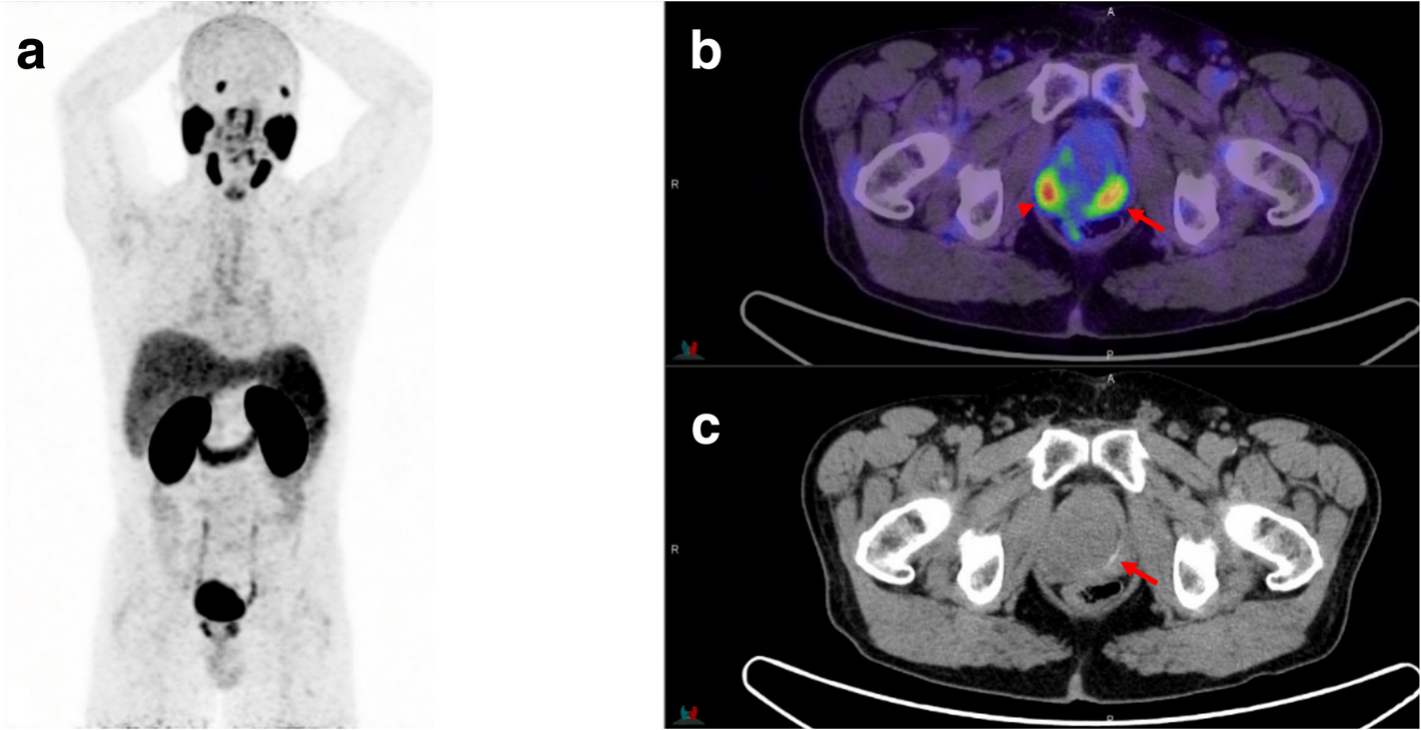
Sixty-year-old man for primary staging of prostate cancer and PSA 18 ng/L. Panel a. PSMA PET MIP image shows two areas of uptake on both sides of the prostate. Panel b and c. On PET/CT-U and CT-U images the left focus (arrow) corresponds to refluxed enhanced urine into prostate capsule and right focus (arrow head) is consistent with prostate cancer. Biopsies of both sides of prostate revealed only right sided malignancy
